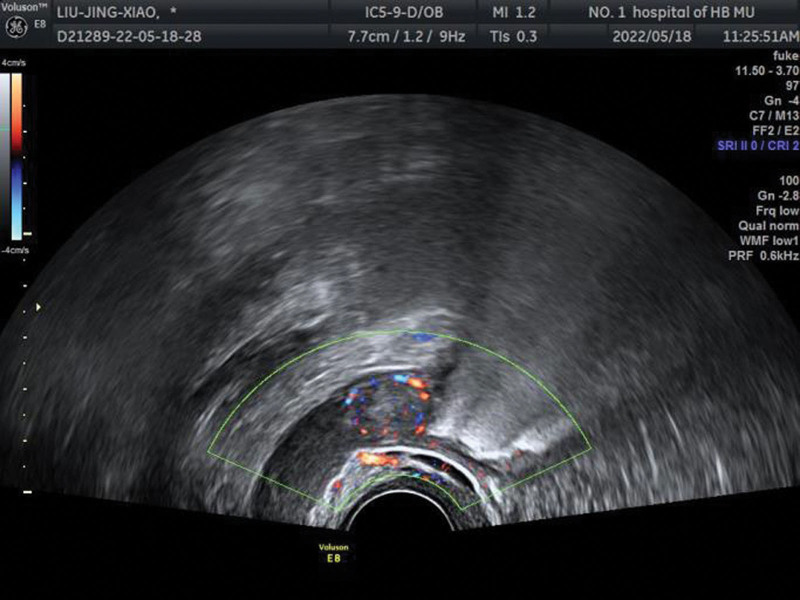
Transvaginal ultrasound: a hypoechoic area in the right ovary, with abundant blood flow around it.
